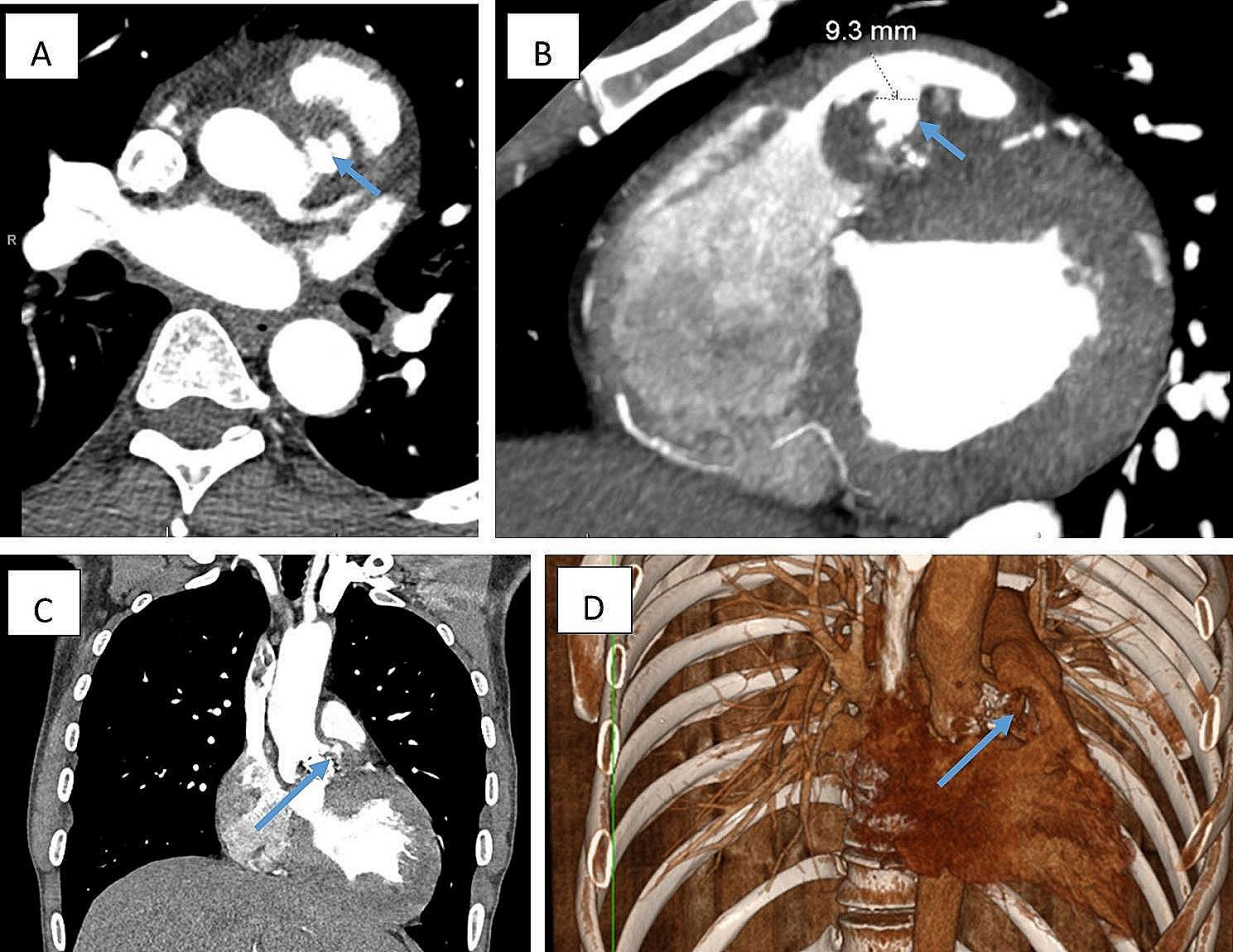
(A) Axial CT of the aorta showing fistula arising from the aortic root outpouching towards the right ventricular outflow tract (RVOT), (B) CT showing the fistulous communication in the RVOT, (C) Coronal CT imaging showing the relationship between the aortic root and the fistula, (D) 3D reconstruction showing the fistula
